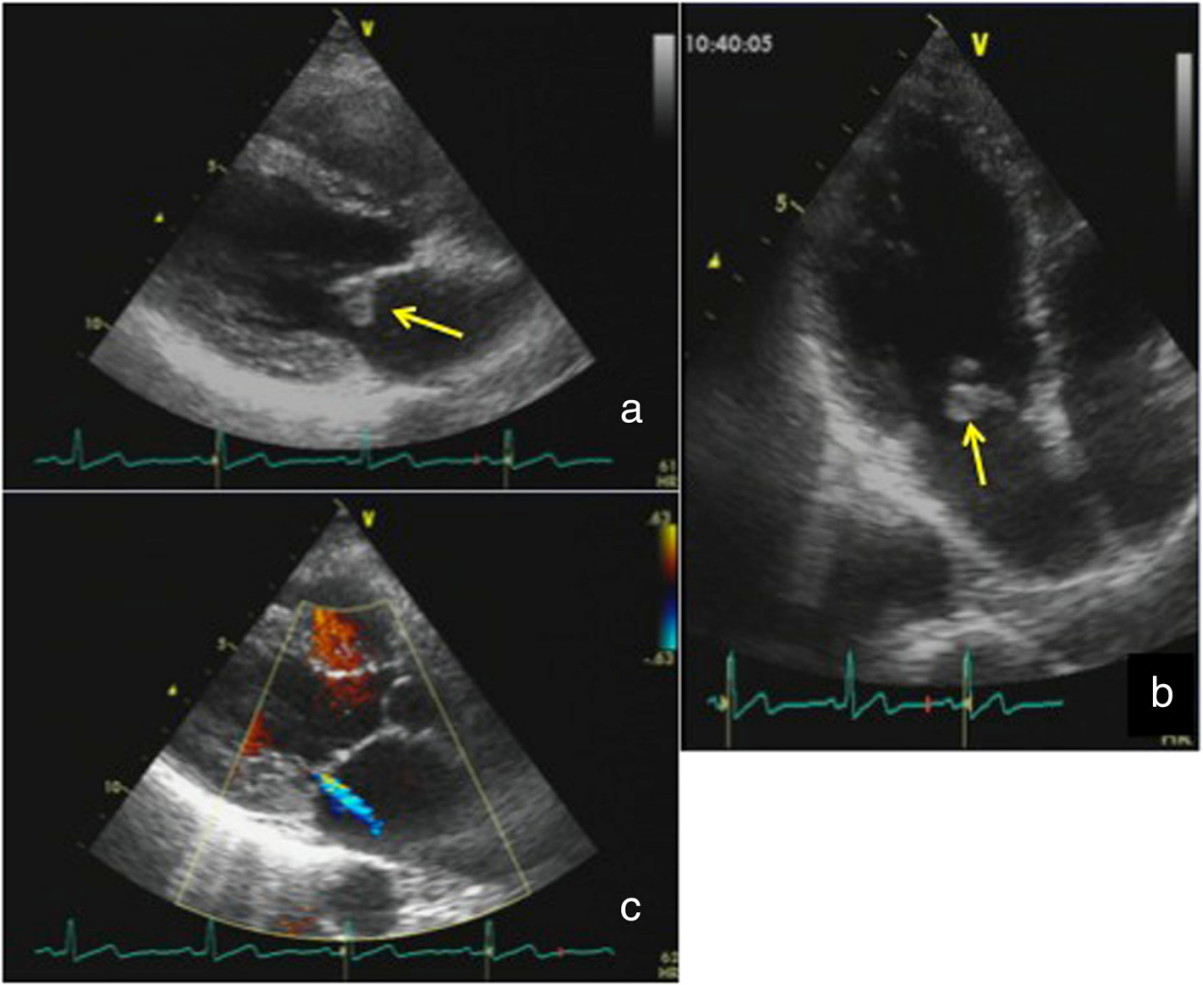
Echocardiographic findings. An 8- × 8-mm tumor-like lesion (↑) was present on the posterior side of the anterior mitral leaflet with trivial regurgitation. a, c Long-axis view. b Four-chamber view
